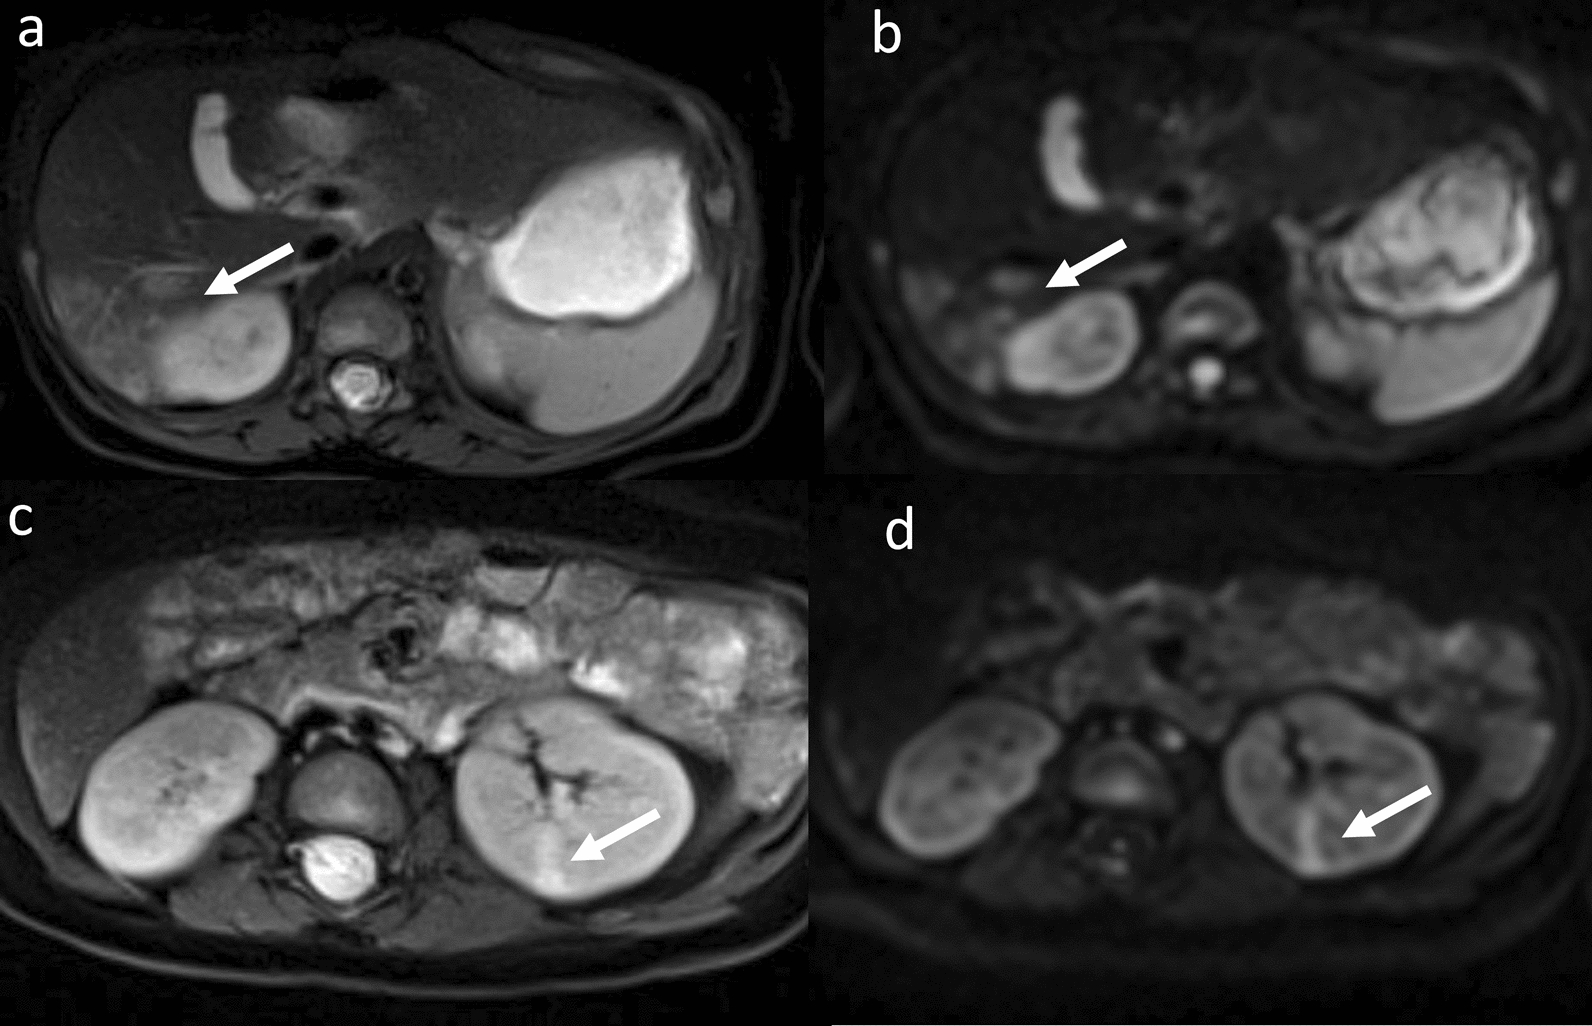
MRI of suspected multifocal vascular malformations in a 11-month-old girl with BWS^UPD^. Arrows indicated multiple patchy hyperintense signals in the liver on the fat-suppressed T2WI (a) and DWI (b). A similar signal was observed in the left kidney on T2WI and DWI (c, d)
